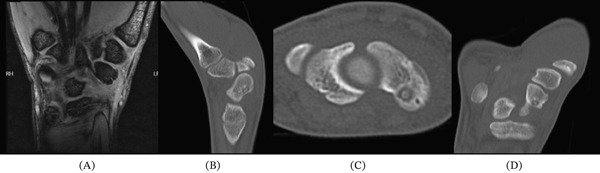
Preoperative MRI and CT scans. (A) Coronal T2‐weighted MRI of the left hand showing increased signal along the radial border of the scaphoid. (B–D) Sagittal, axial, and coronal CT images of the left wrist demonstrating a scaphoid nidus with surrounding sclerosis.
